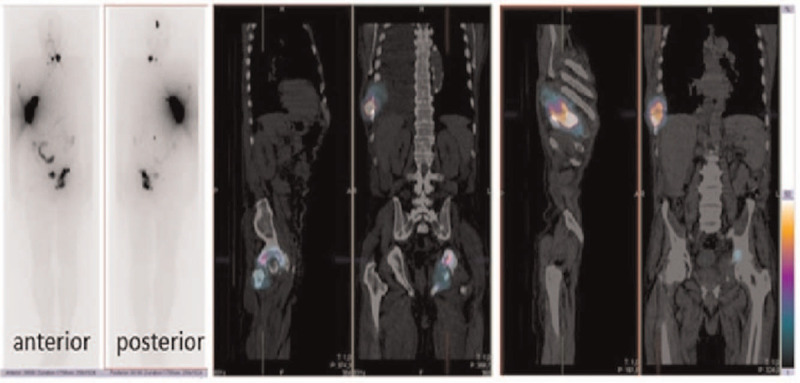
SPECT/CT with iodine-123 (January 2018) after total thyroidectomy showed a large pathologic accumulation in the 8th right rib and additional pathologic accumulations in the thyroid remnant, on the left side of the neck, in the left acetabulum, in the skull and in the right humerus.
